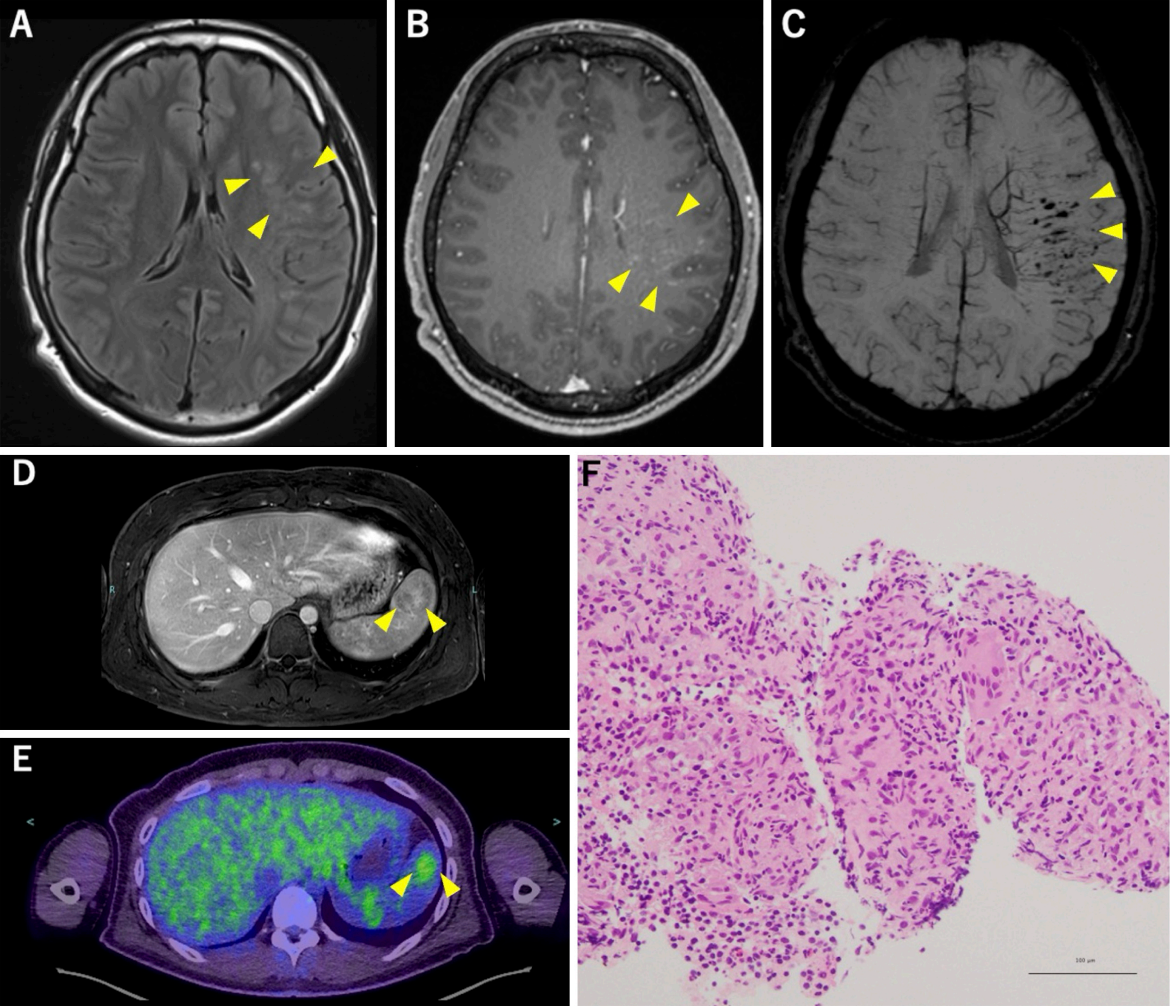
Brain MRI reveals hyperintense lesions in the left inferior frontal gyrus, superior temporal gyrus, and supramarginal gyrus on axial FLAIR (A, yellow arrowheads), and gadolinium-enhanced T1-weighted imaging shows linear contrast enhancement along medullary veins (B, yellow arrowheads). Susceptibility-weighted imaging reveals dilated medullary veins and multiple hypointense areas indicative of microbleeds (C, yellow arrowheads). Abdominal MRI reveals mild splenomegaly and a focal lesion with heterogeneous contrast enhancement during portal venous phase (D, yellow arrowheads), and ^18^F-FDG-PET/CT shows abnormal uptake corresponding to this splenic region, with a an SUV max of 3.1 (E, yellow arrowheads). A splenic biopsy confirms clusters of epithelioid cells without caseous necrosis (F). ^18^F-FDG-PET/CT: ^18^F-fluorodeoxyglucose positron emission tomography/computed tomography; FLAIR: fluid-attenuated inversion recovery; MRI: magnetic resonance imaging; SUV max: maximal standardized uptake value.
